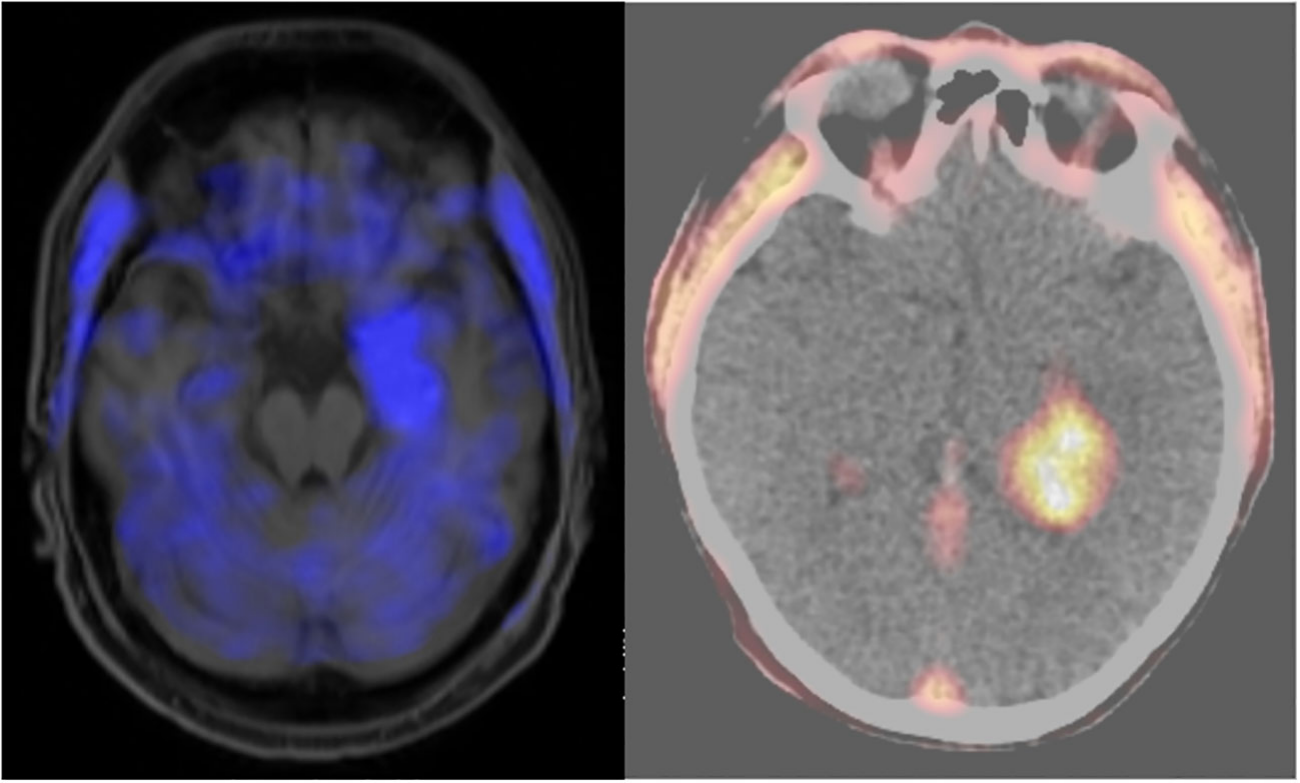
18F FET PET imaging from to the two cases shown in Figure 1. Left: PET MRI image corresponding to the MRI imaging planes shown in the upper panels of Figure 1. There was slightly positive tracer uptake in the non-MRI contrast-enhancing tumor (the mean tumor-to-background ratio (TBR) was 1.6, with a normal TBR being <1.6), indicating a possible high-grade tumor. Right: PET CT image corresponding to the MRI imaging planes shown in the lower panels of Figure 1. There is high tracer uptake in the MRI contrast-enhancing tumor, supporting the suspicion of a high-grade tumor (TBR = 3.1). Both patients had glioblastoma.
